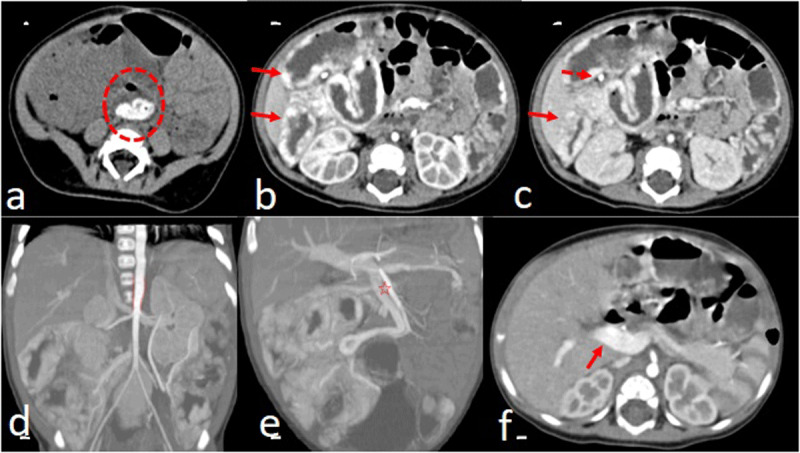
Abdominal CT images – a) axial pre-contrast; b) axial arterial phase; c) axial portal phase; d) coronal MIP arterial phase; e) coronal MIP portal phase; f) axial arterial phase at the level of the portal vein – showed high-density material in the rectum consistent with blood (dashed circle in a) and thickening of the colon wall with intense globular mural enhancement and diffuse filling (arrows in b and c). The mesentery of the right quadrants was also thickened and enhancing, and a small mesenteric phlebolith (dashed arrow in c) was seen. MIP images showed tapering of the aorta at the level of the superior mesenteric artery (faded lines in d) and enlargement of the superior mesenteric artery and vein (star in e). The portal vein was also enlarged and showed avid enhancement in the arterial phase (arrow in f).
